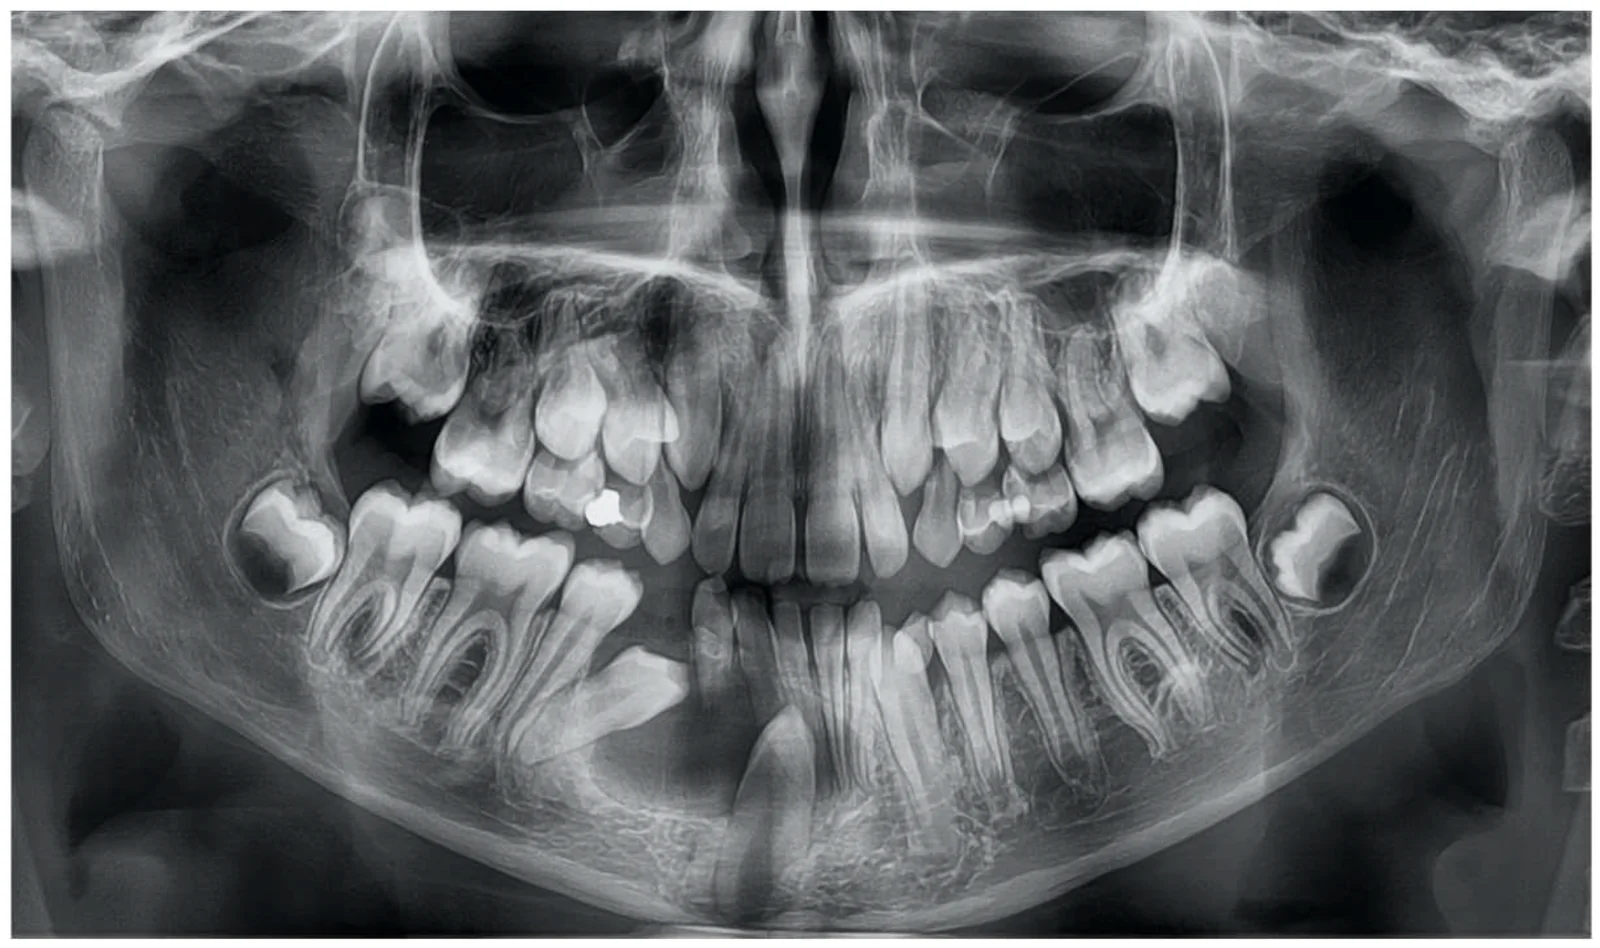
Initial panoramic radiograph.
